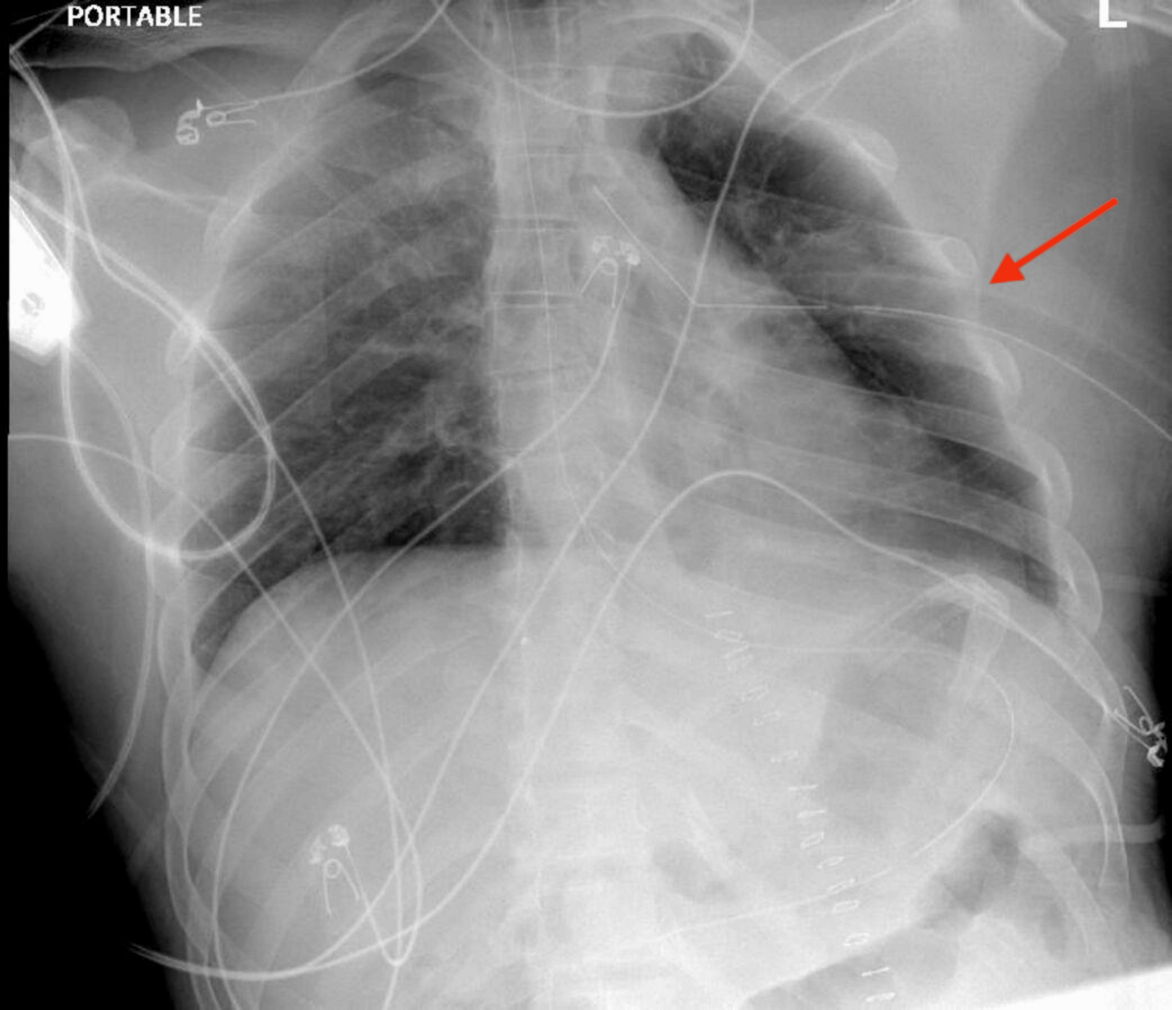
Chest X-ray showing the left chest tube properly placed to evacuate left hemothorax (red arrow).
